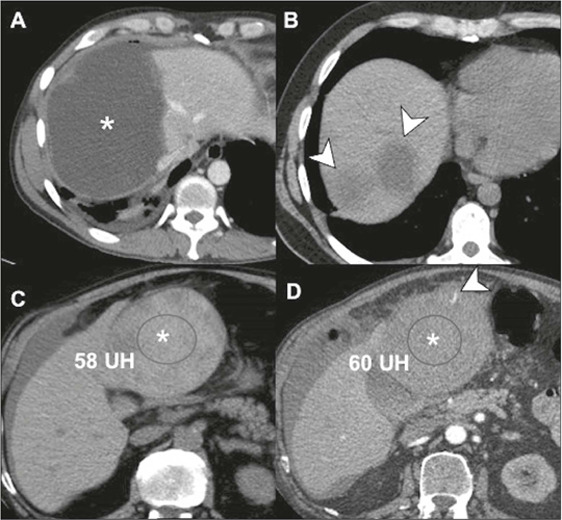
CT scans of cysts of traumatic origin. A: Biloma. A
